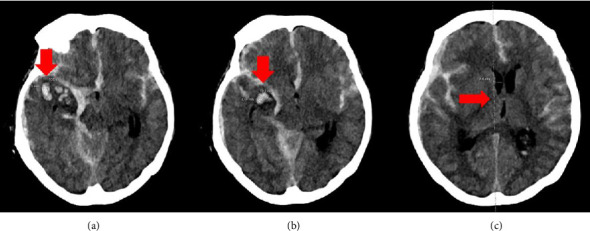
Subarachnoid hemorrhage and intraventricular hemorrhage and leftward midline shift. (a, b) Head CT demonstrating diffuse subarachnoid hemorrhage and a 4 × 2 × 2 mm right posterior communicating artery aneurysm. (c) Head CT showing diffuse cerebral edema with a 9 mm leftward midline shift, effacement of the suprasellar cistern, and medialization of the right uncus, concerning for developing descending transtentorial herniation.
